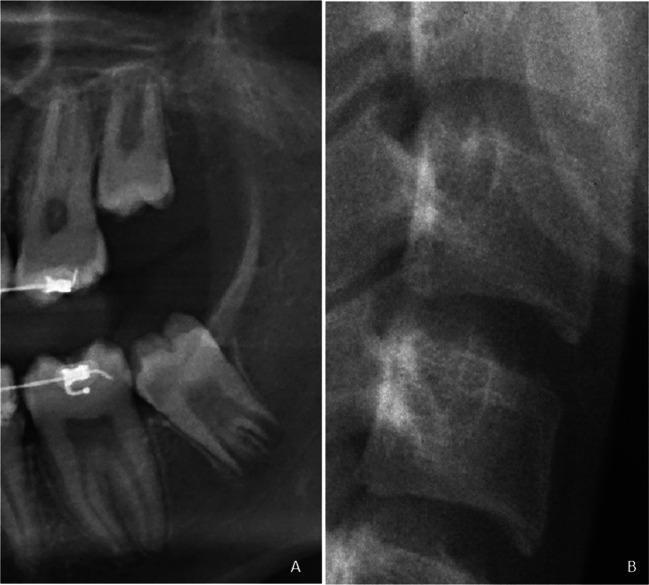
Example of a male individual with chronological age 17.34 years. The cropped panoramic radiograph (A) shows the left third molars and the cropped cephalometric radiograph (B) shows the inferior surfaces of cervical vertebrae C2, C3, and C4. The maxillary third molar was classified as stage F1 and the mandibular third molar was classified as stage F1. The anterior inferior apophysis ossification stages of cervical vertebrae C2, C3, and C4 were classified as stage 1,2,2 respectively
